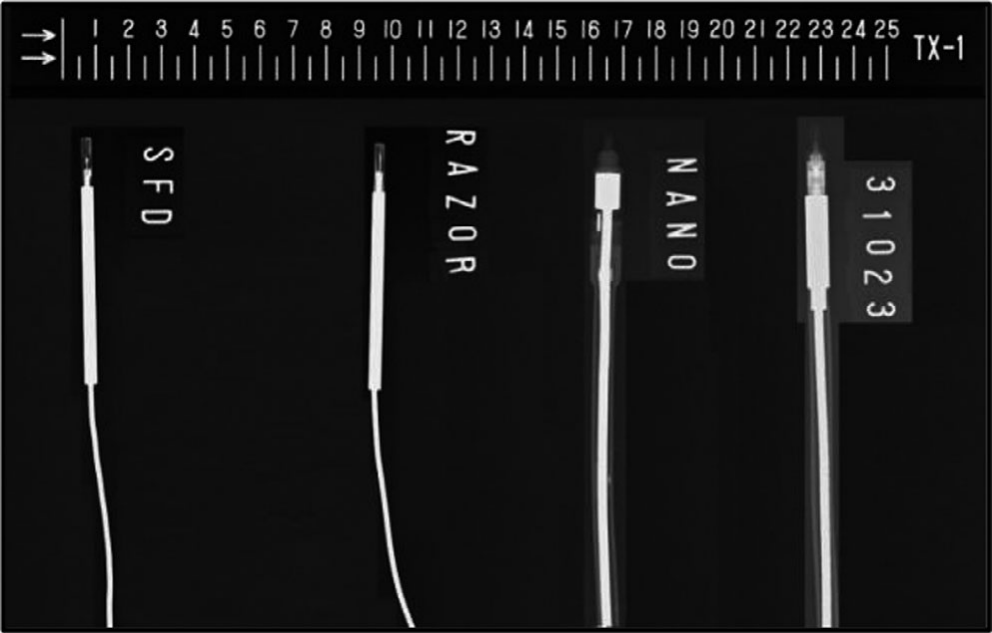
Radiograph of small field detectors used in this work (left to right) stereotactic field diode, RAZOR Diode, RAZOR Nanochamber, and PTW31023. The scale above is graduated in cm.
